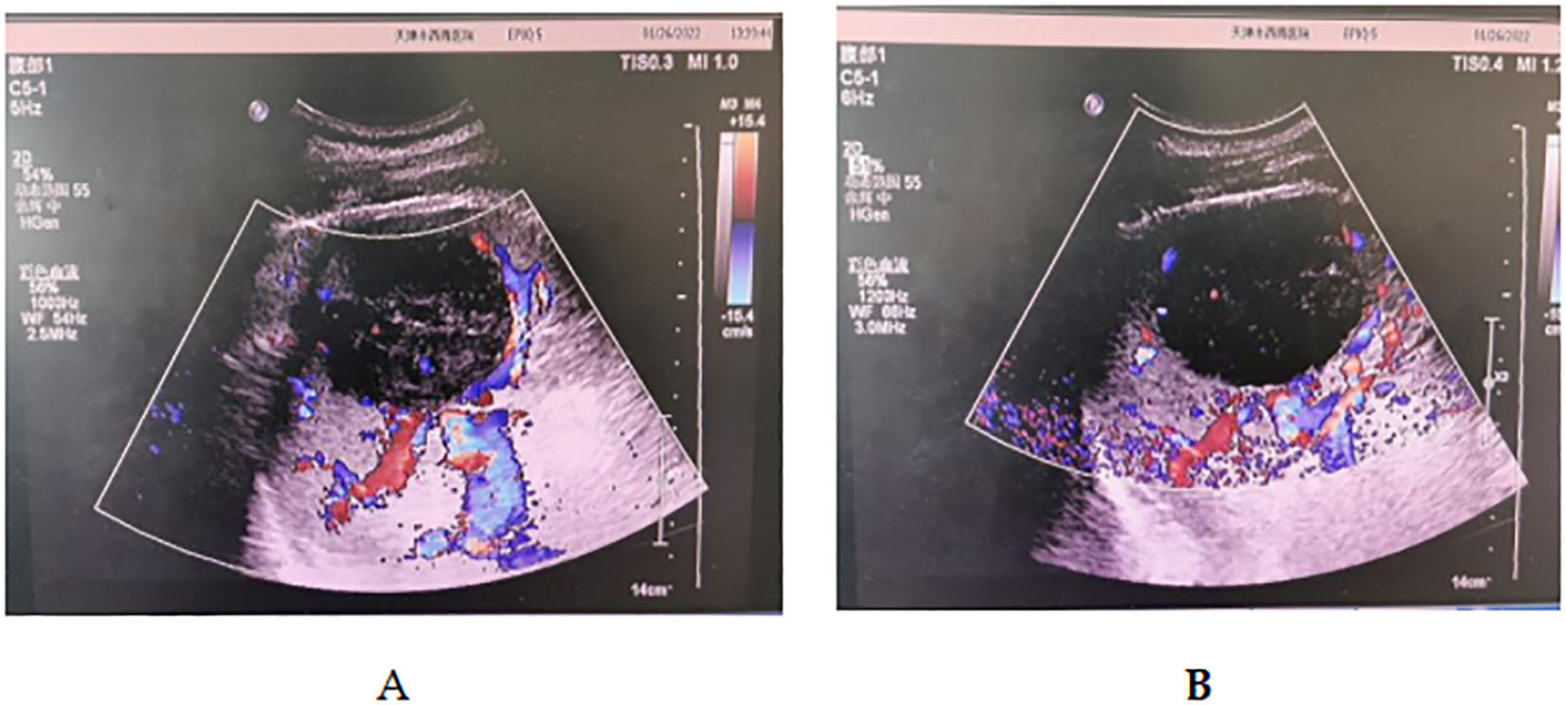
(A, B) Ultrasound imaging revealed a 7.8 x 6 cm hypoechoic mass within the spleen.
